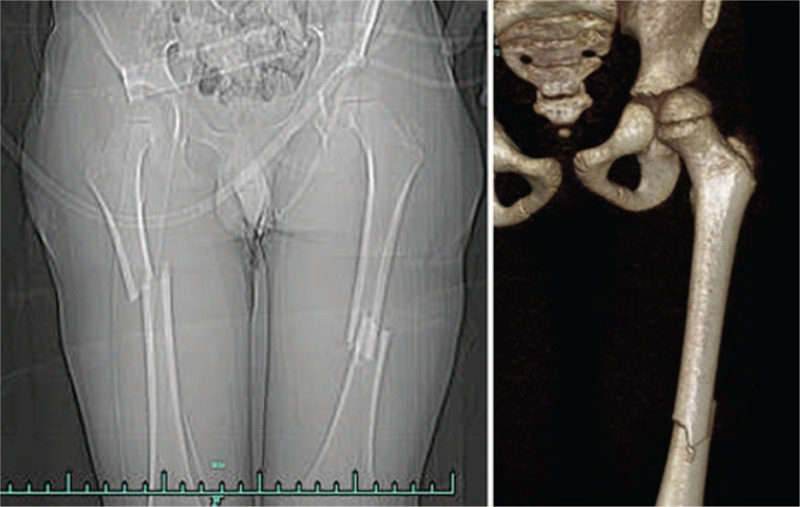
Preoperative three-dimensional CT examination of the femur can clearly observe the femoral fracture line.
